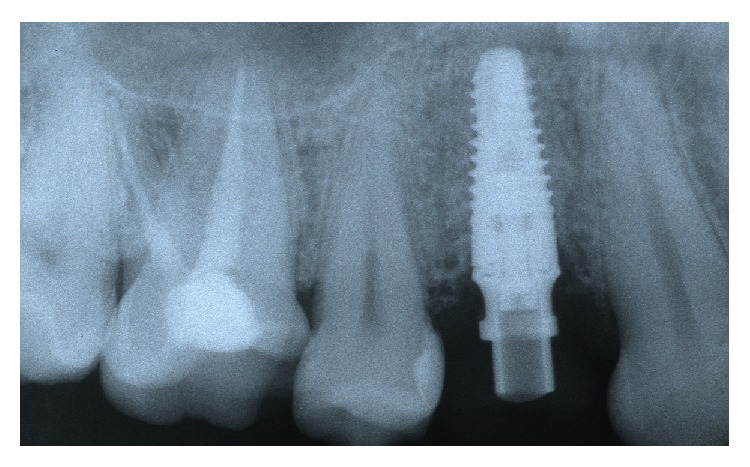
Periapical Rx of temporary screw rehabilitation.
